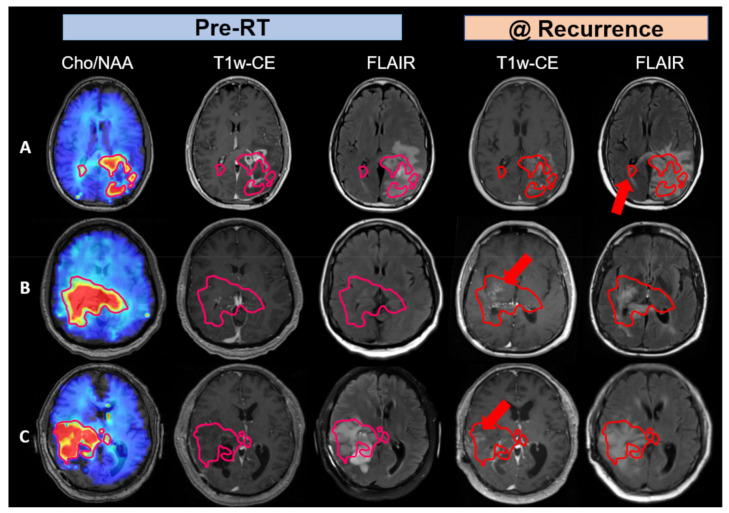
Pre-RT sMRI scans suggest out-of-field recurrence in the belinostat cohort with tumor infiltration beyond what is shown in standard imaging (indicated by red arrows). (A) This patient had two-fold Cho/NAA elevation that had spread contralaterally. While pre-RT standard imaging failed to detect this, FLAIR hyperintensity at recurrence mimics the direction of tumor infiltration detected by pre-RT sMRI. (B) The second patient had tumor infiltration across the midline that wasn’t detected by pre-RT standard imaging. Lesions at recurrence in both T1w-CE and FLAIR MRIs confirm Cho/NAA abnormalities in the pre-RT sMRI scan, suggesting standard imaging underestimated the extent of tumor infiltration. (C) This patient had a large lesion of metabolically active tumor that was not shown in T1w-CE, thus, undertreated, which became apparent at recurrence.
